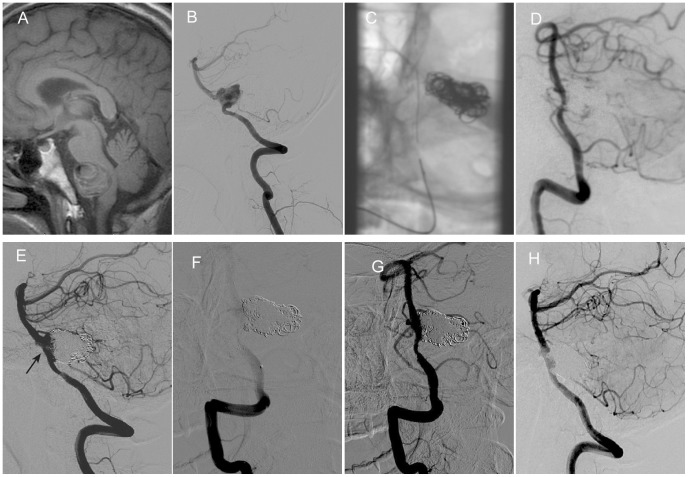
Retreatment with coil embolization was performed for recurrent intracranial VADA after SAC. A 29-year old male presented with dizziness and hemiplegia. Sagittal view of MR imaging showed intramural hematoma and compression to brain stem (A). Right vertebral angiograms showed a dissecting aneurysm involving PICA (B). SAC (C) were performed with nearly complete occlusion (D). Follow up angiography after three months revealed recanalization of the dissecting aneurysm (black arrow) (E). Retreatment was performed by coil embolization (F, G). Follow up angiography after 7 months of retreatment showed complete occlusion of right vertebral dissecting aneurysm (H).
